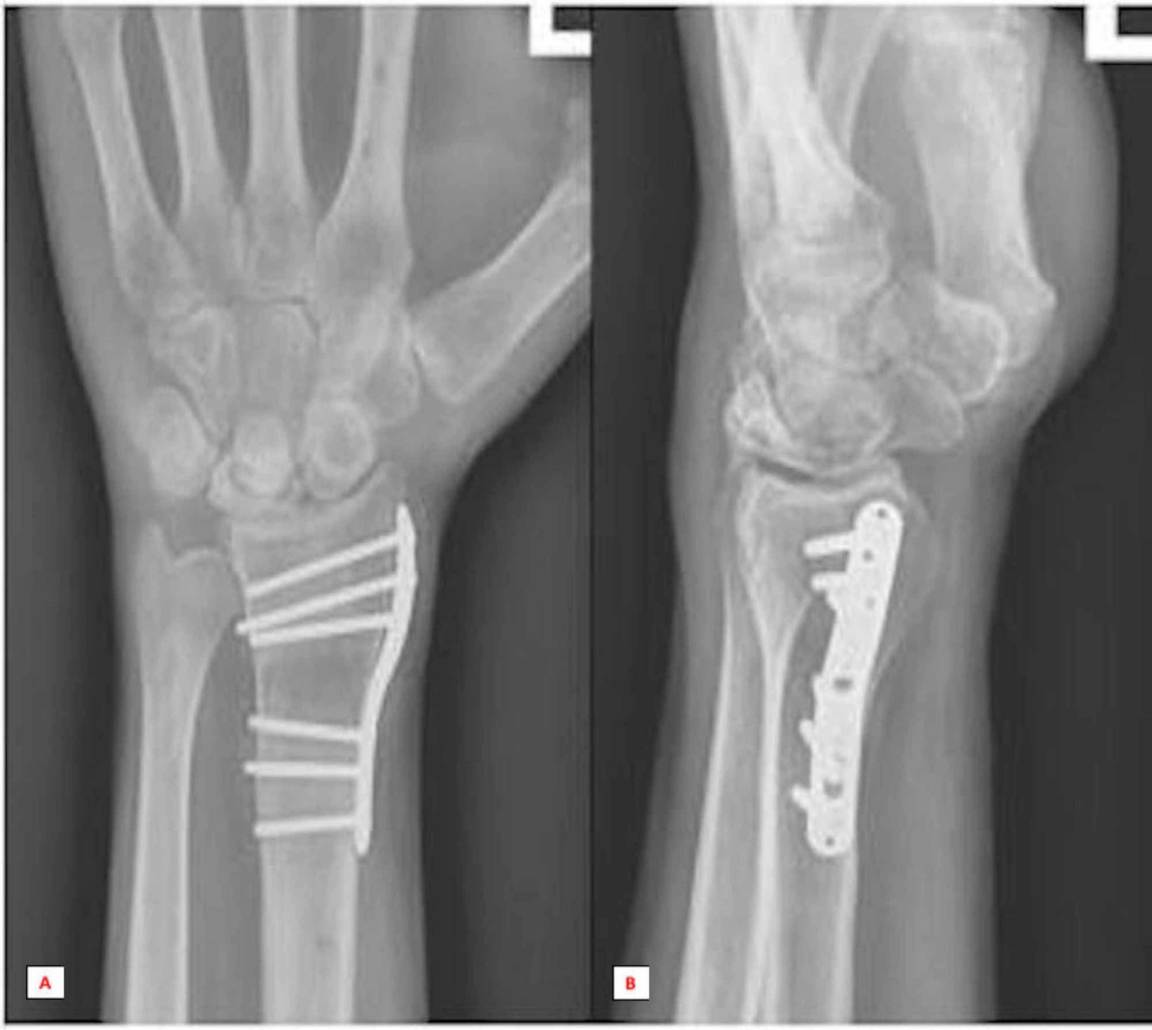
Anterior-posterior (A) and lateral (B) radiographic series at six months post-surgical follow-up revealed the complete collapse of the lunate.
